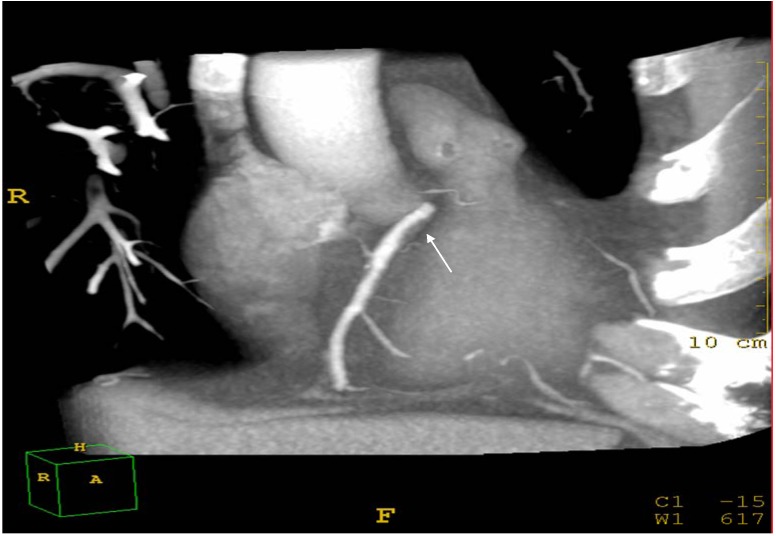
Cardiac CT: Example of soft (non calcified) coronary plaques at the proximal area of the right coronary artery (arrow).
